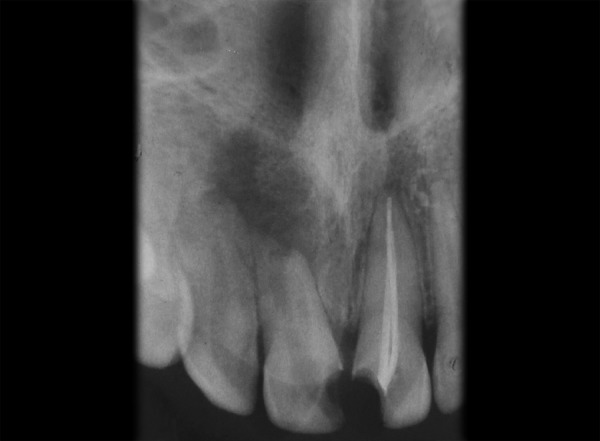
Maxillary right incisor of a 15-year-old male showing an infection induced communicating internal-external inflammatory root resorption and a related periapical inflammatory lesion in 11
